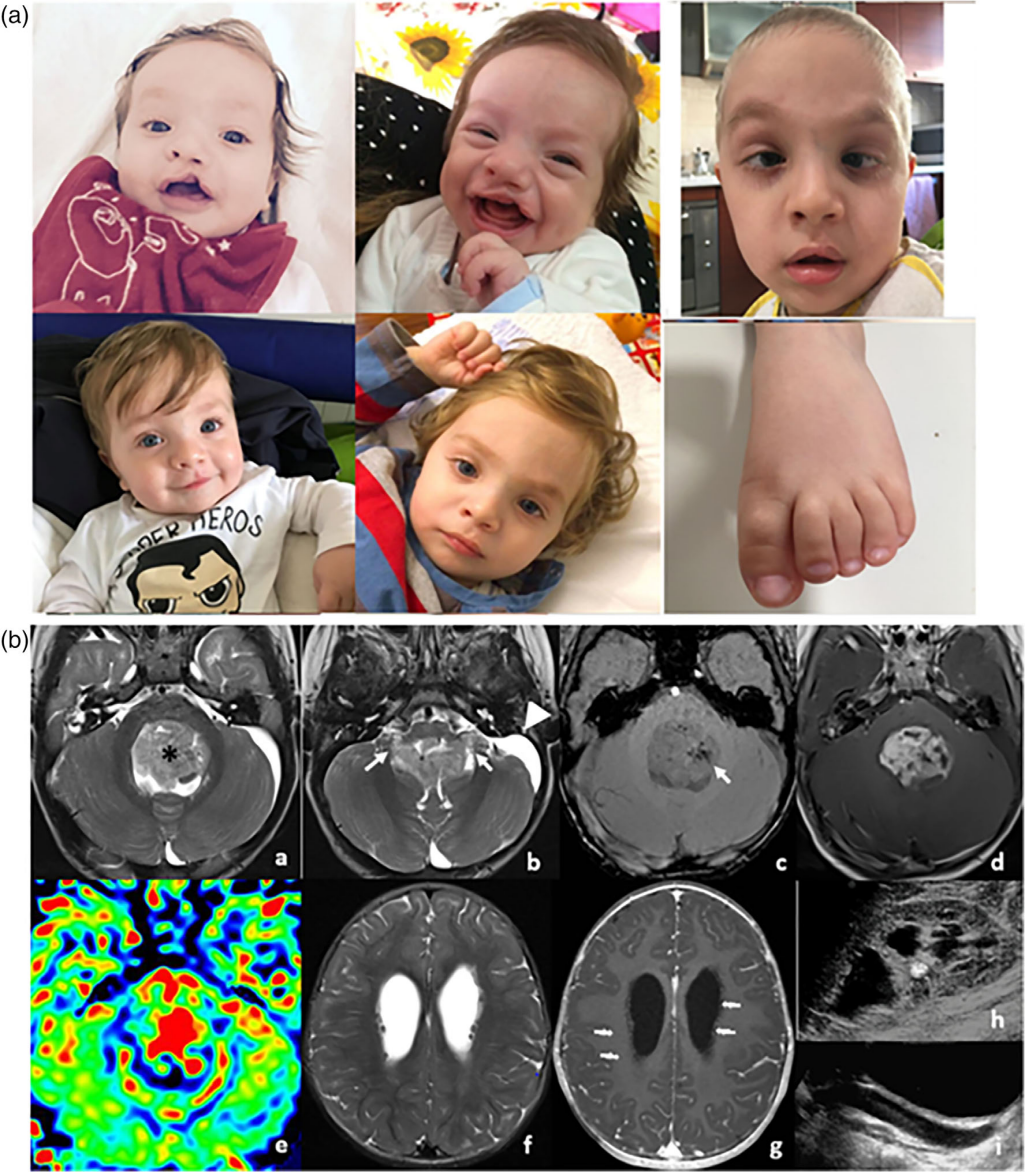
(a) Patient's facial appearance at different ages; left foot with partial cutaneous syndactyly between the 2nd and 3rd toes. (b) Posterior fossa ependymoma. Magnetic resonance imaging (MRI), T2‐weighted (T2w, a, b), susceptibility‐weighted imaging (SWI, c) gadolinium‐enhanced T1‐weighted (GdT1w, d) axial images. Fourth ventricular mass (*) shows heterogeneous T2 hyperintensity (a, b), extension through the foramina of Luschka (b, arrows), punctate hypointense foci (c, arrow) consistent with intratumoral calcifications, predominantly solid enhancement on GdT1w images (d), and elevated perfusion in the cerebral blood volume (CBV) map (e). Note enlargement of the pericerebellar cisternal space on the left with scalloping of the bone, suggestive of an arachnoid cyst (b, arrowhead). Multiple, bilateral periventricular nodular heterotopia. T2w (f) and GdT1w (g) axial MRI images show bilateral heterotopic nodules isointense to gray matter on both T2w and T1w images without contrast‐enhancement, causing distortion of the ventricular margins (arrows). Renal involvement. Ultrasound examination of the right kidney reveals hydroureteronephrosis and lithiasis (h, i)
